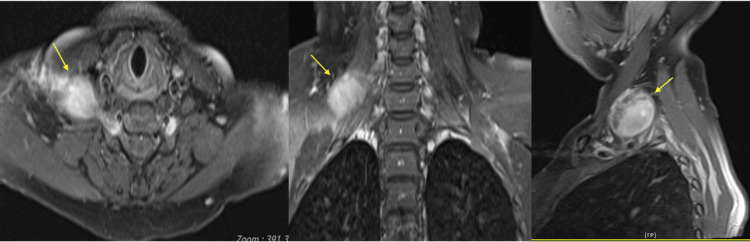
T2-weighted MRI of the neck with contrast demonstrates heterogeneously hyperintense lesion at the right cervical paravertebral region (yellow arrow).
